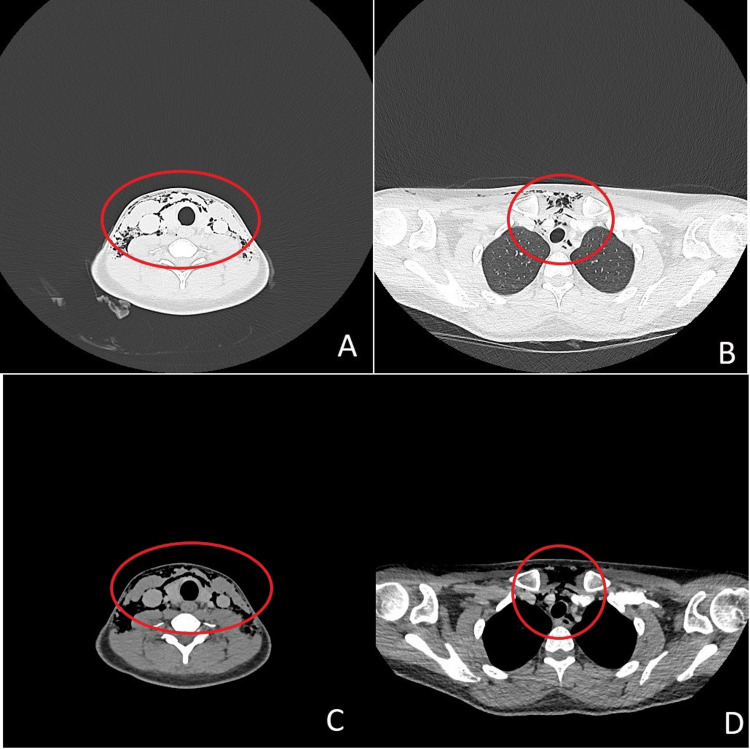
CT scan of the first episode showing subcutaneous emphysema in the neck and pneumomediastinum (A) Neck imaging in lung window, (B) thoracic imaging in lung window, (C) neck imaging in soft tissue window, and (D) thoracic imaging in soft tissue window CT: computed tomography
